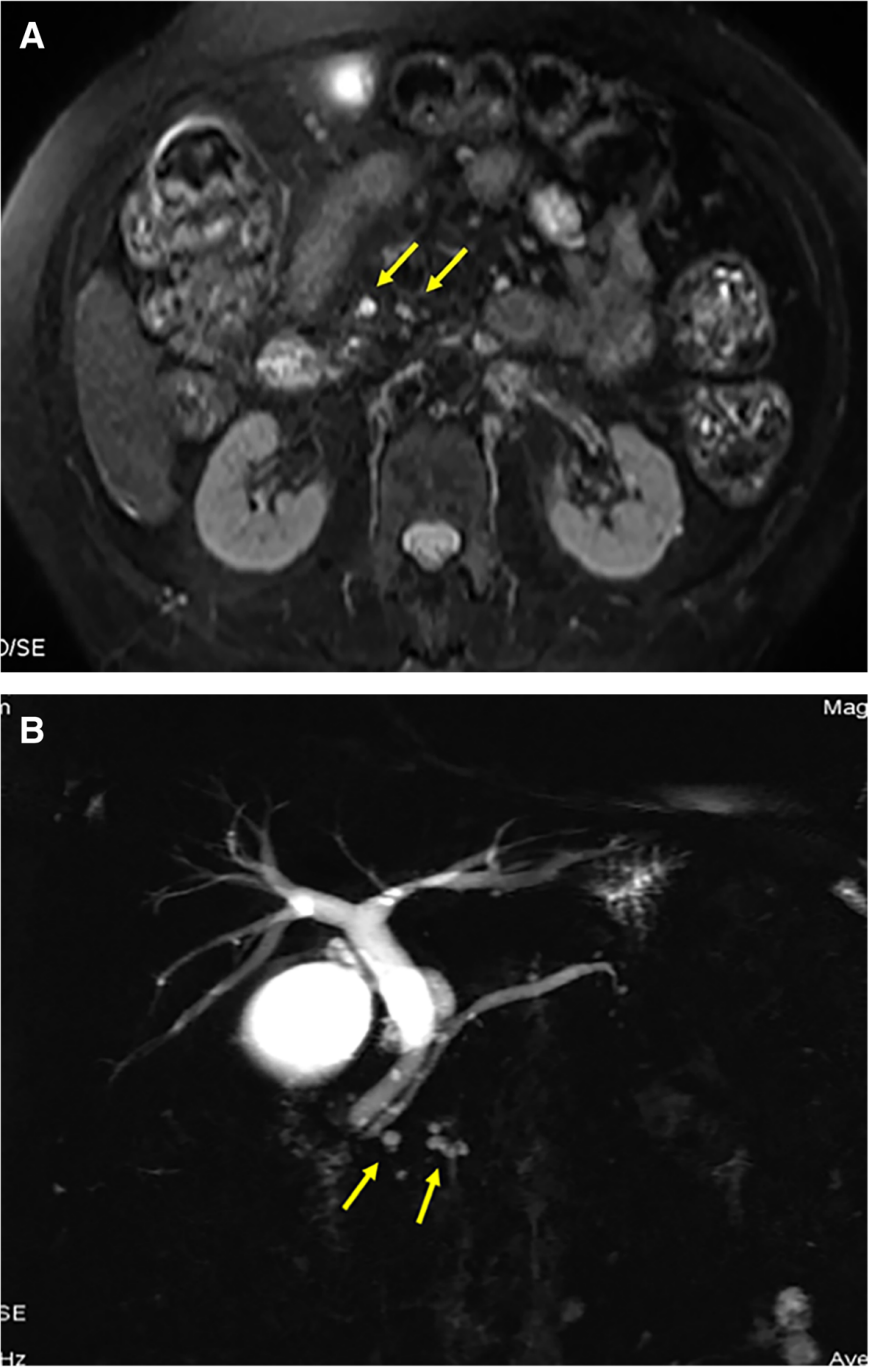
Imaging features of PCLs detected by MRI. A patient with multiple small cysts in the head of pancreas. a, and b, transverse T2-weighted fat-suppressed turbo-spin-echo (TSE) MR images and MR cholangiopancreatography show multiple cysts (arrow) with well-defined homogeneous signal intensity in the head of pancreas
